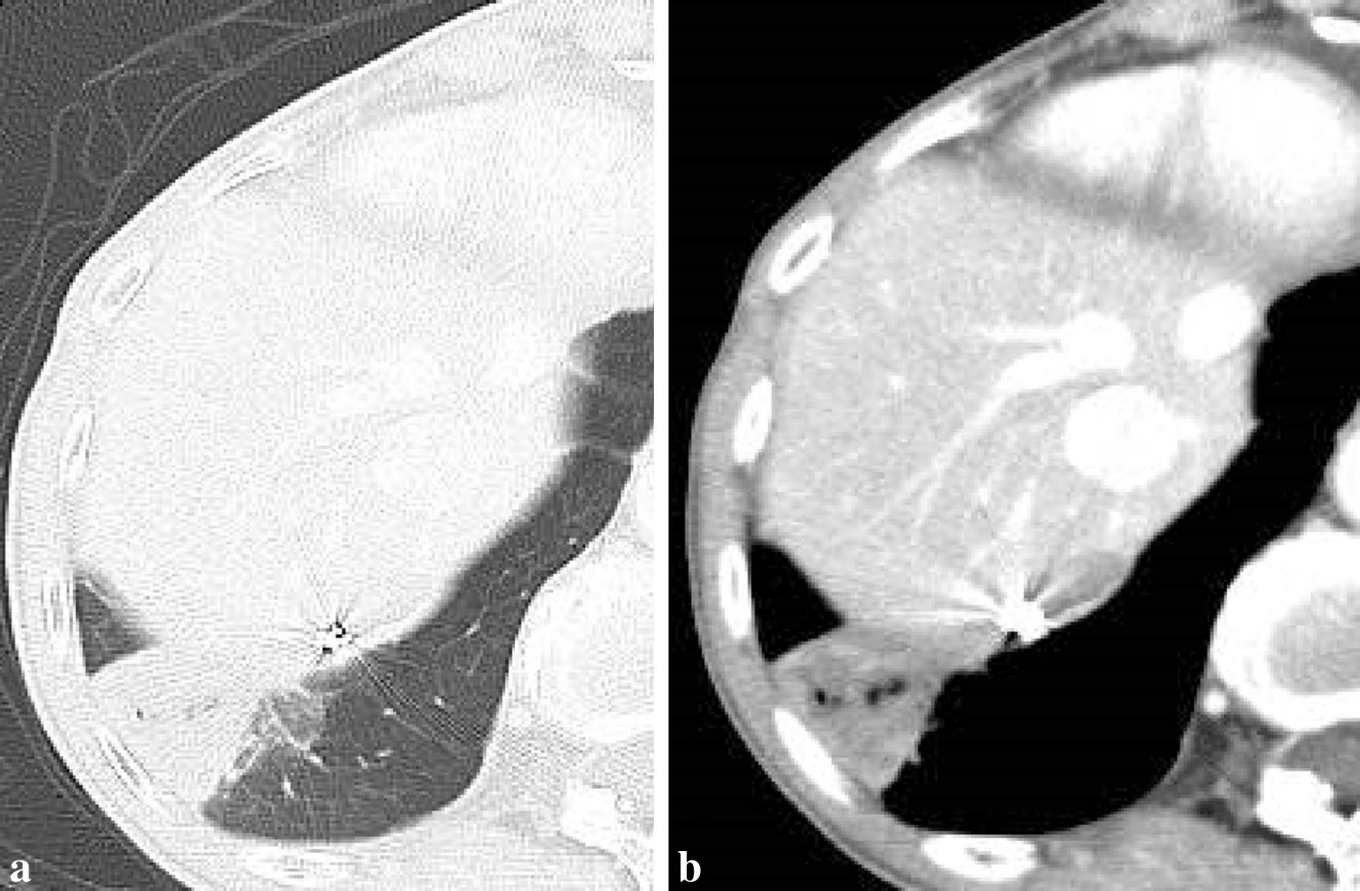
Contrast-enhanced computed tomography showed a localized wedge-shaped pulmonary opacity closely approximating the pleura, which indicated pulmonary infarction. a lung window image, b mediastinal window image
